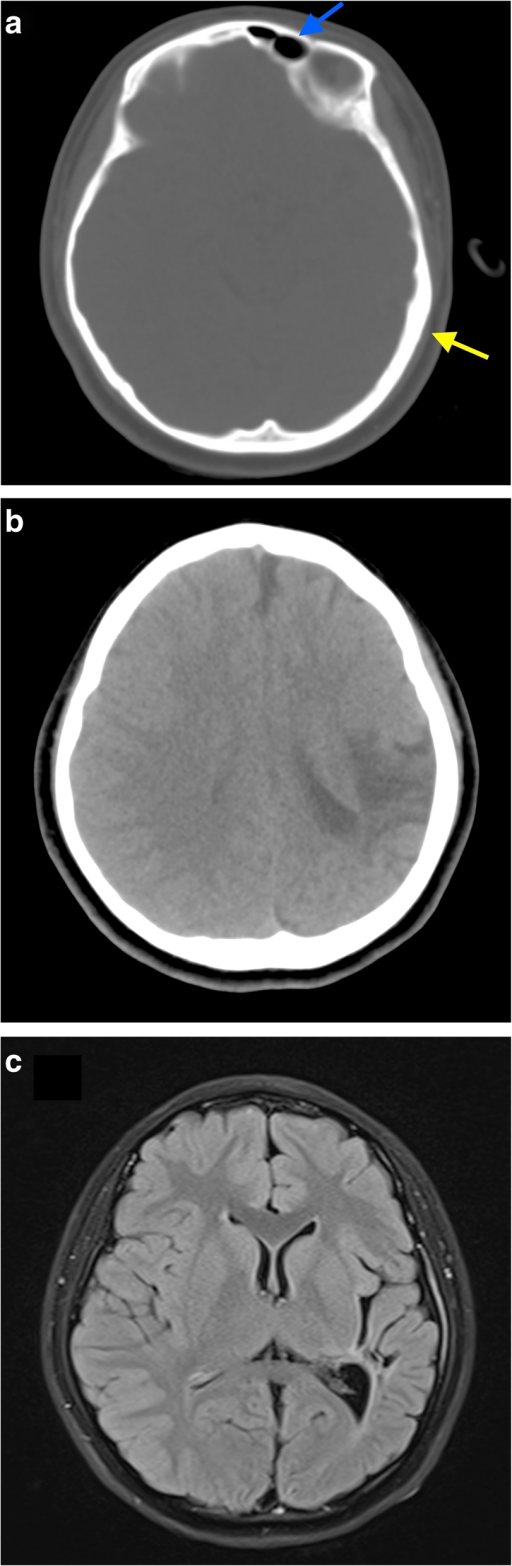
CT (a, b) and MRI (c) images, in axial section showing. a CT image in bone window showing hyperpneumatization of the left frontal sinus (blue arrow) with compensatory calvarial thickening (yellow arrow). b CT image (non-contrasted) showing the left parieto-temporal infarct. c MRI T2 FLAIR image showing left cerebral hemiatrophy, with dilated left lateral ventricle. Focal encephalomalacia and gliotic changes are also noted
